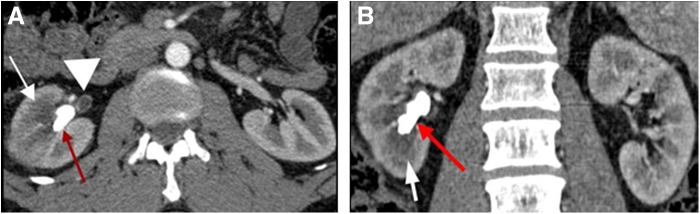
Preoperative axial (A) and coronal (B) arterial phase CT images at the level of the kidneys showing partial staghorn calculus in the right collecting system (*red arrows*). There is heterogeneous enhancement of the right kidney (*white arrows*) radiographically consistent with pyelonephritis and abnormal proximal right ureteral enhancement (*white arrowhead*) radiographically consistent with ureteritis.
